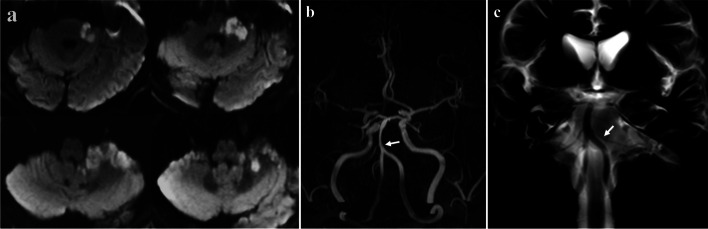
Diffusion-weighted image revealed left anterior inferior cerebellar artery (AICA) territorial infarction (a).On magnetic resonance angiography, the left AICA was absent, while the right AICA was visualized clearly (b). Basi-parallel anatomic scanning magnetic resonance imaging (BPAS MRI) showed normal bilateral AICA (c)
